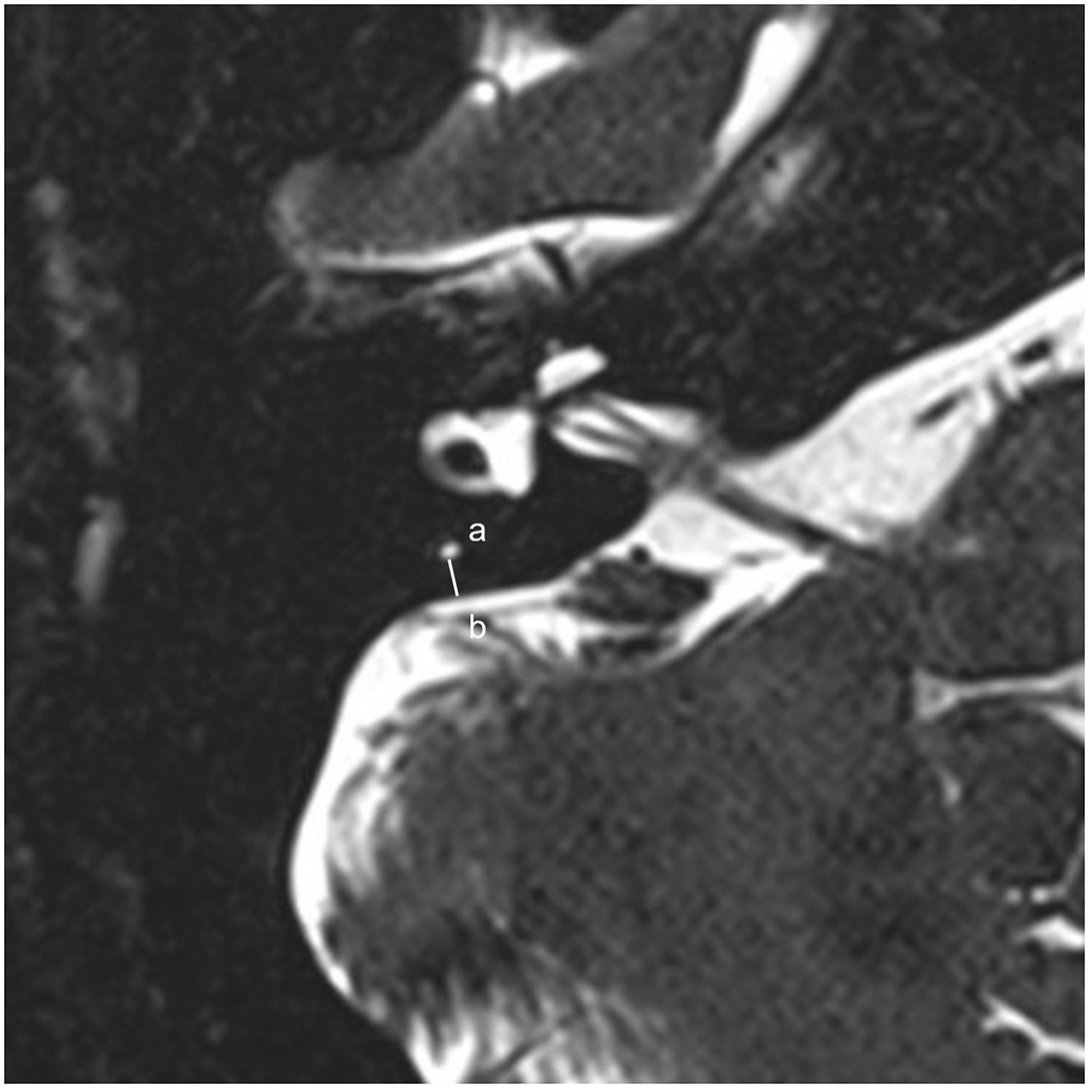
A 0.5-mm axial 3D-SPACE MRI scan showing detailed image of the right ear at the level of the measured distance between the vertical part of the posterior semicircular canal (a) and the posterior fossa (b). *3D-SPACE*, three-dimensional sampling perfection with application optimized contrasts using different flip angle evolutions.
